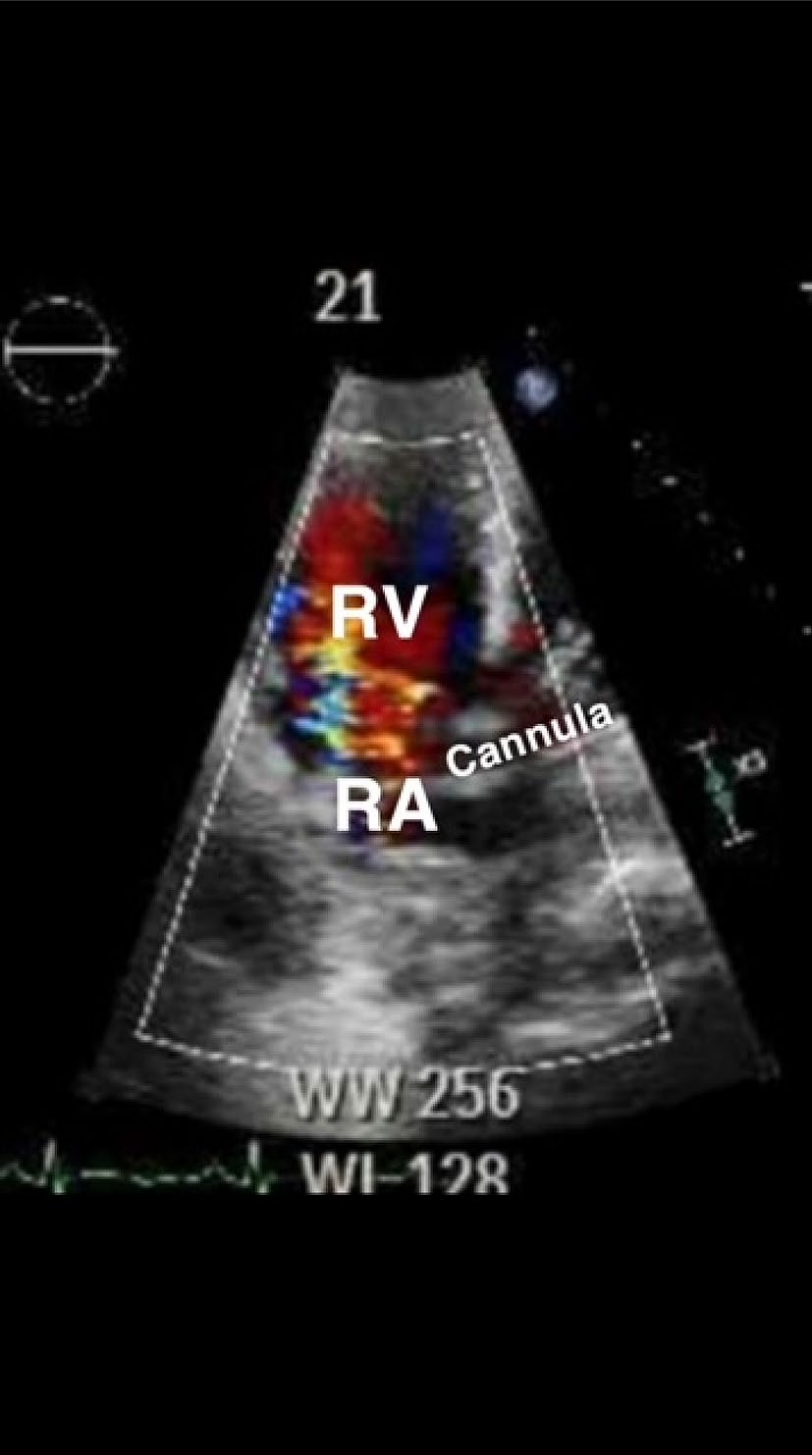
Parasternal right ventricular (RV) inflow view showing the return jet appropriately positioned in the RV
